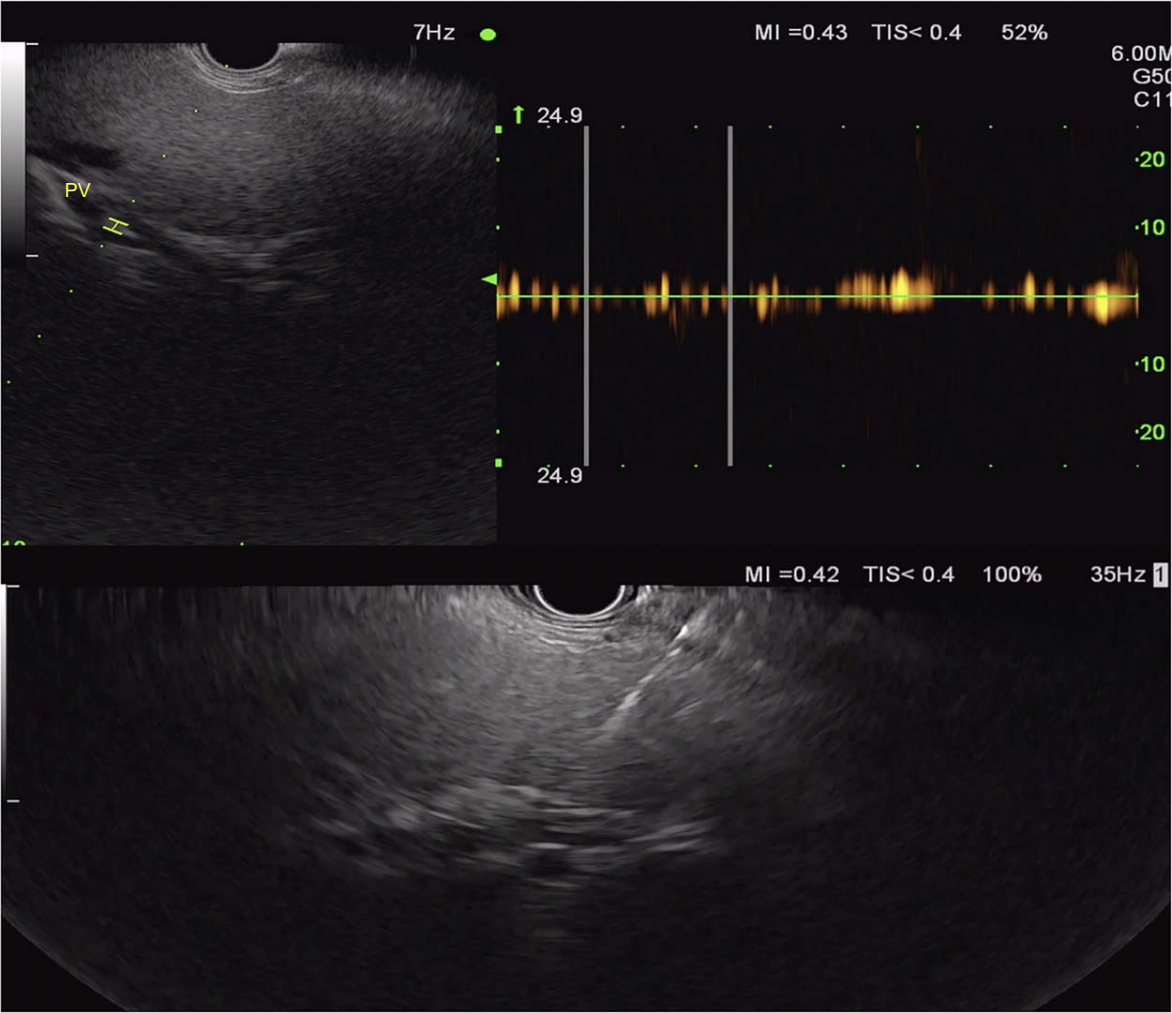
EUS-guided portal pressure measurement of the portal vein. Abbreviation: EUS, endoscopic ultrasound.
